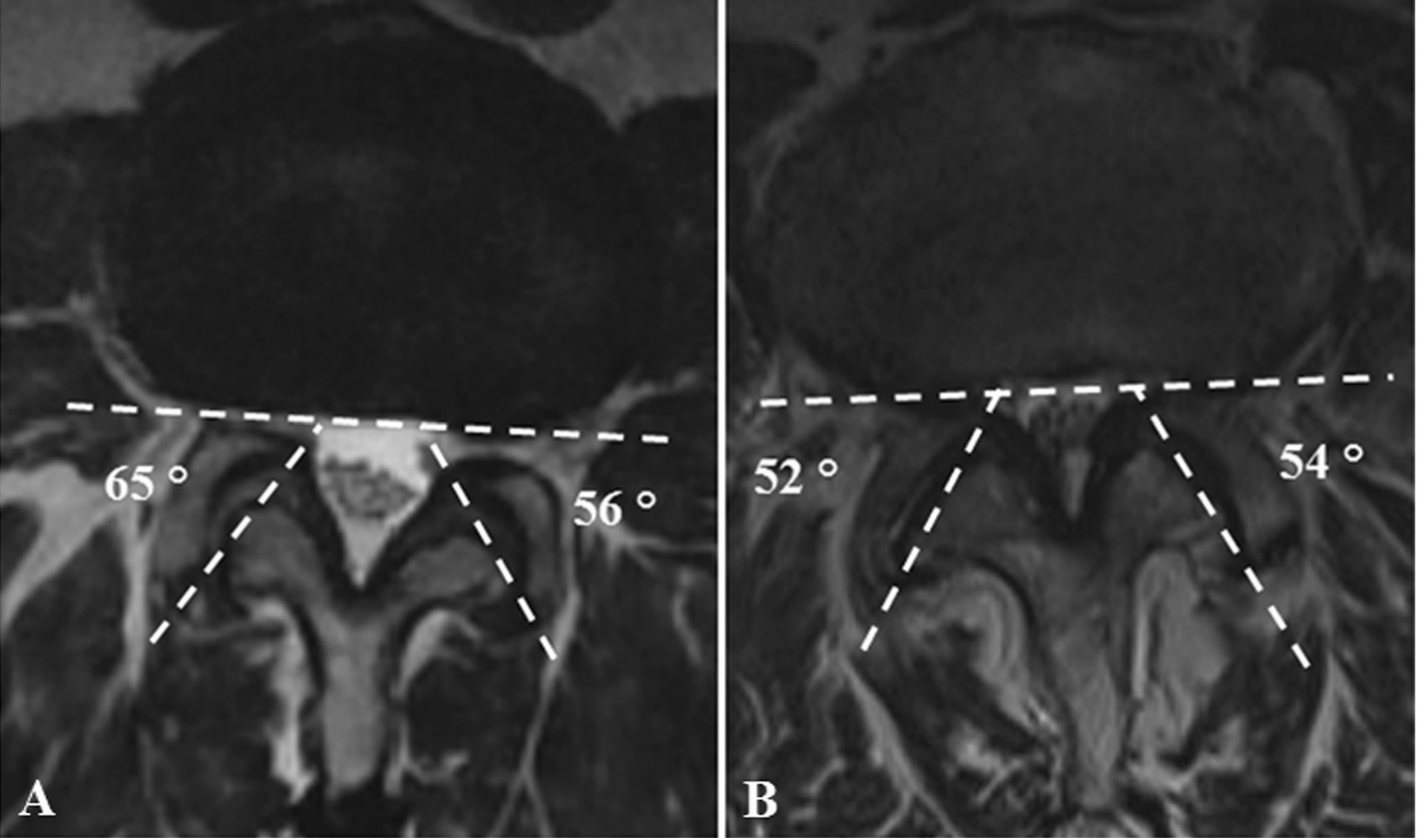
Axial MRI noting assessment of facet joint angulation. The images note patients with facet joint a) tropism and b) non-tropism. Asymmetry of the left and right facet joint angulations greater or equal to 8° angulation was defined as tropism
